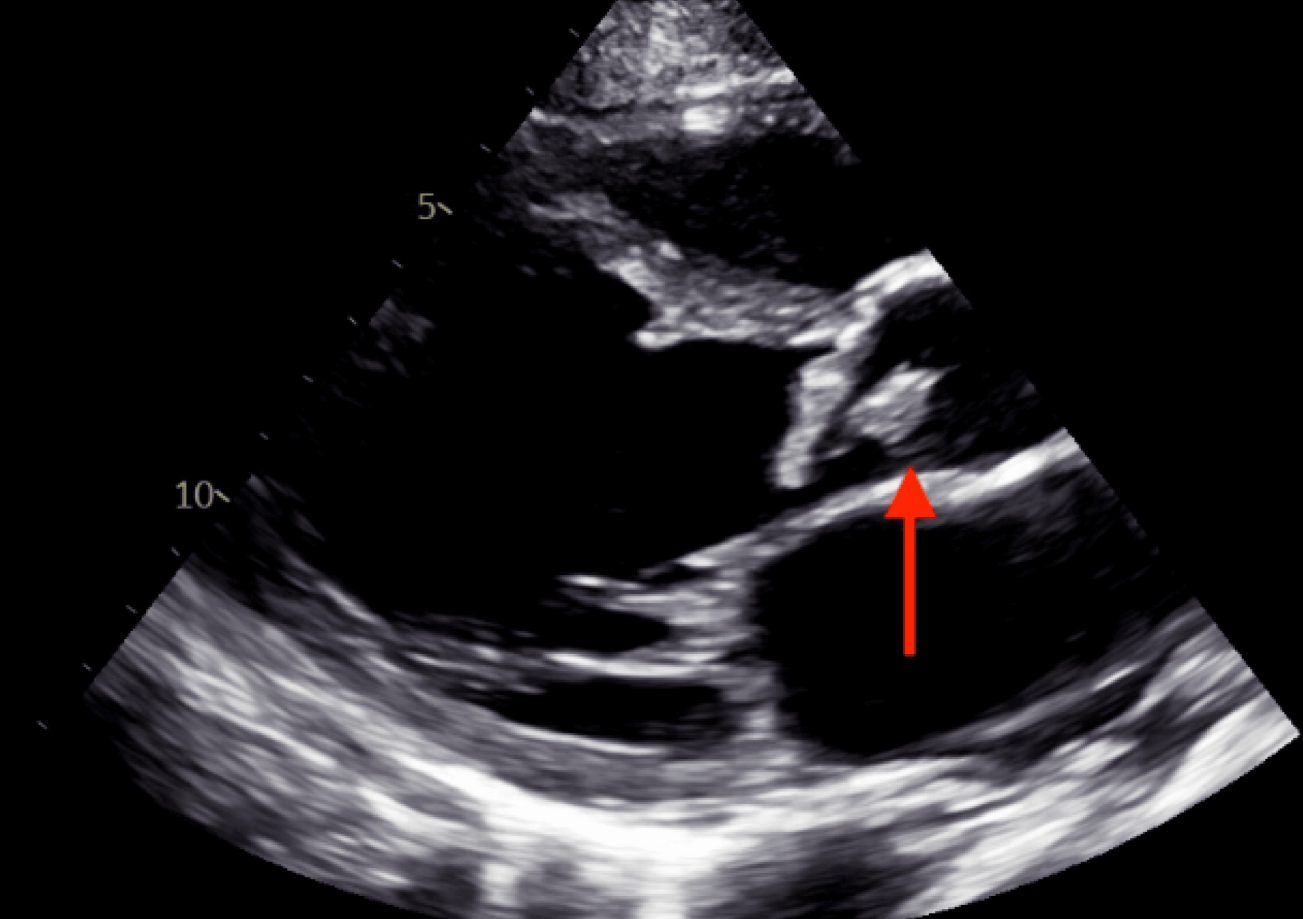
TTE with a parasternal long-axis view. The red arrow points to a mobile, echogenic mass attached to the valve leaflet suggestive of aortic valve vegetation. TTE: Transthoracic echocardiogram
